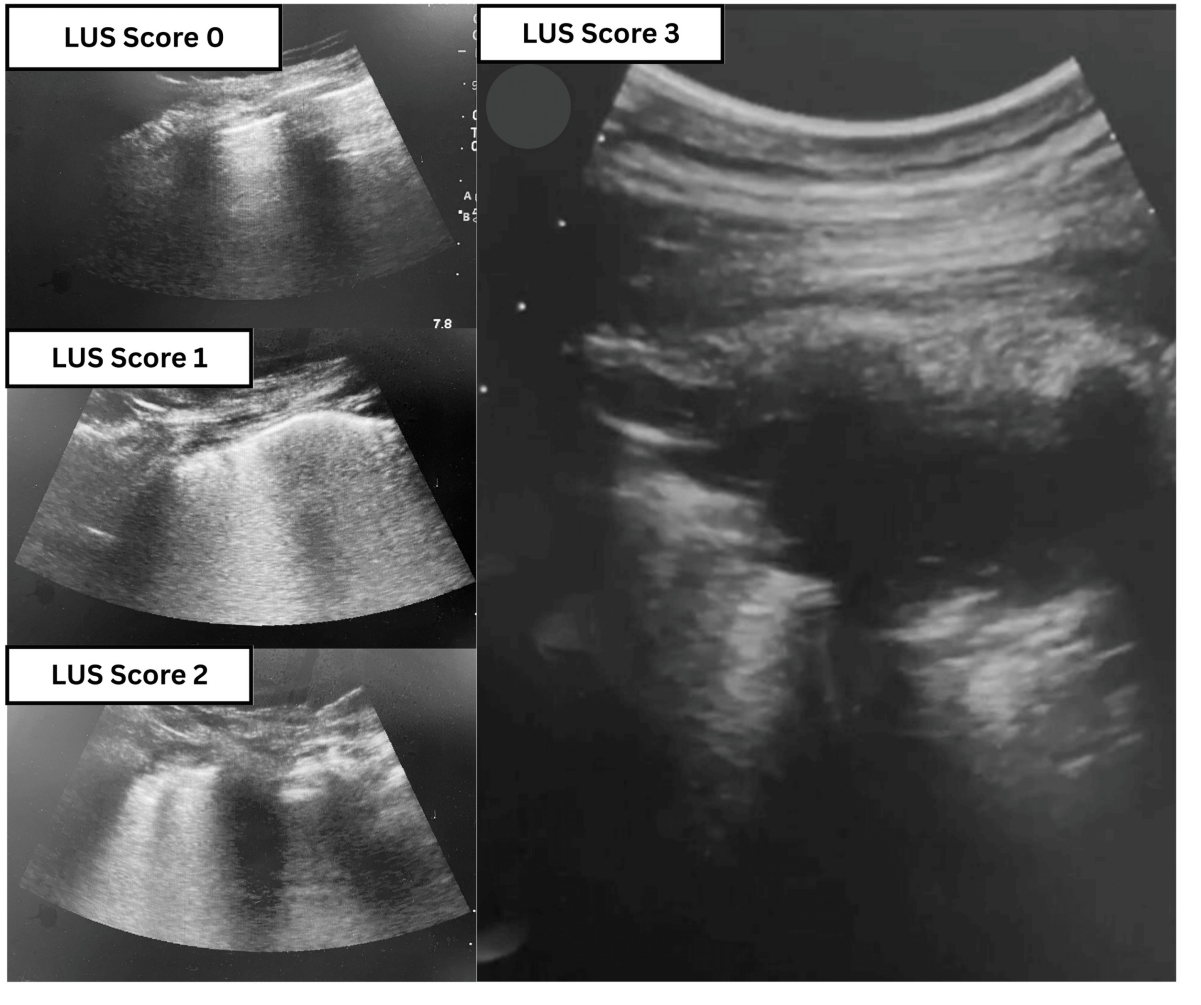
Classification of lung atelectasis severity using lung ultrasound. Severity of atelectasis using Lung ultrasound.
